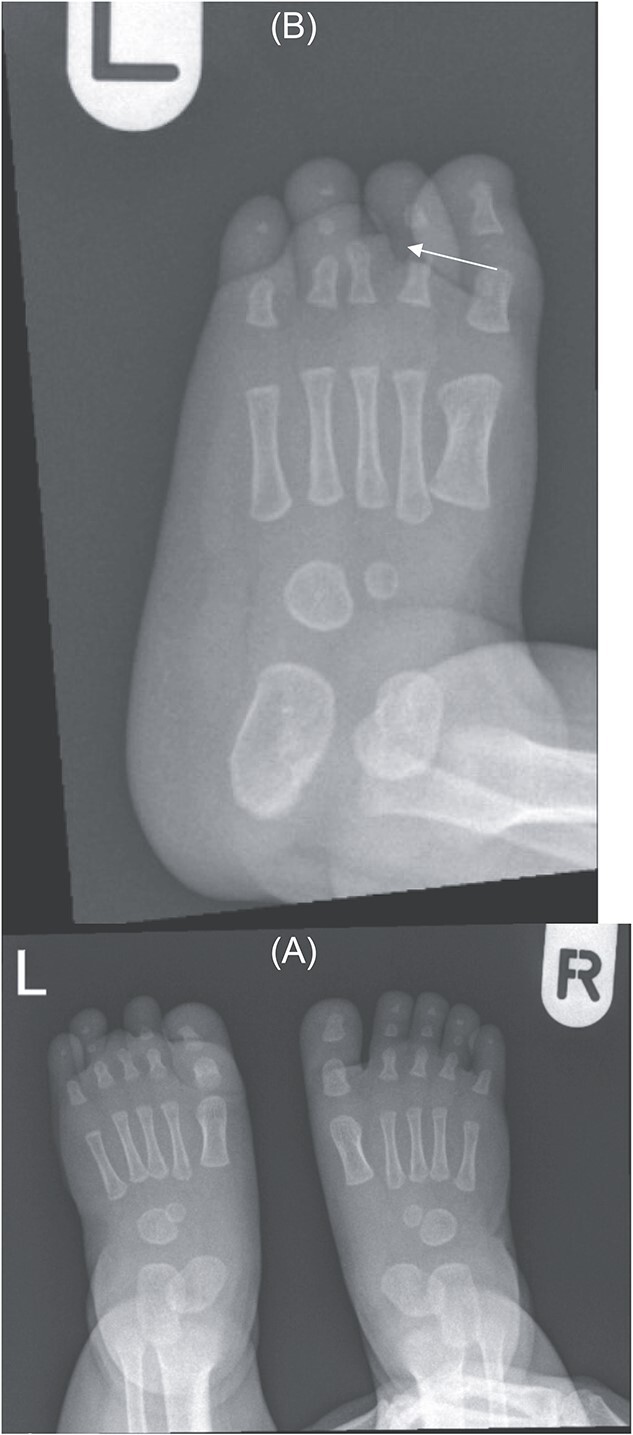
(A) Bilateral feet X-ray with normal right foot bone anatomy and left foot X ray. (B) No visualization of third toe middle and distal phalanges (arrow).
